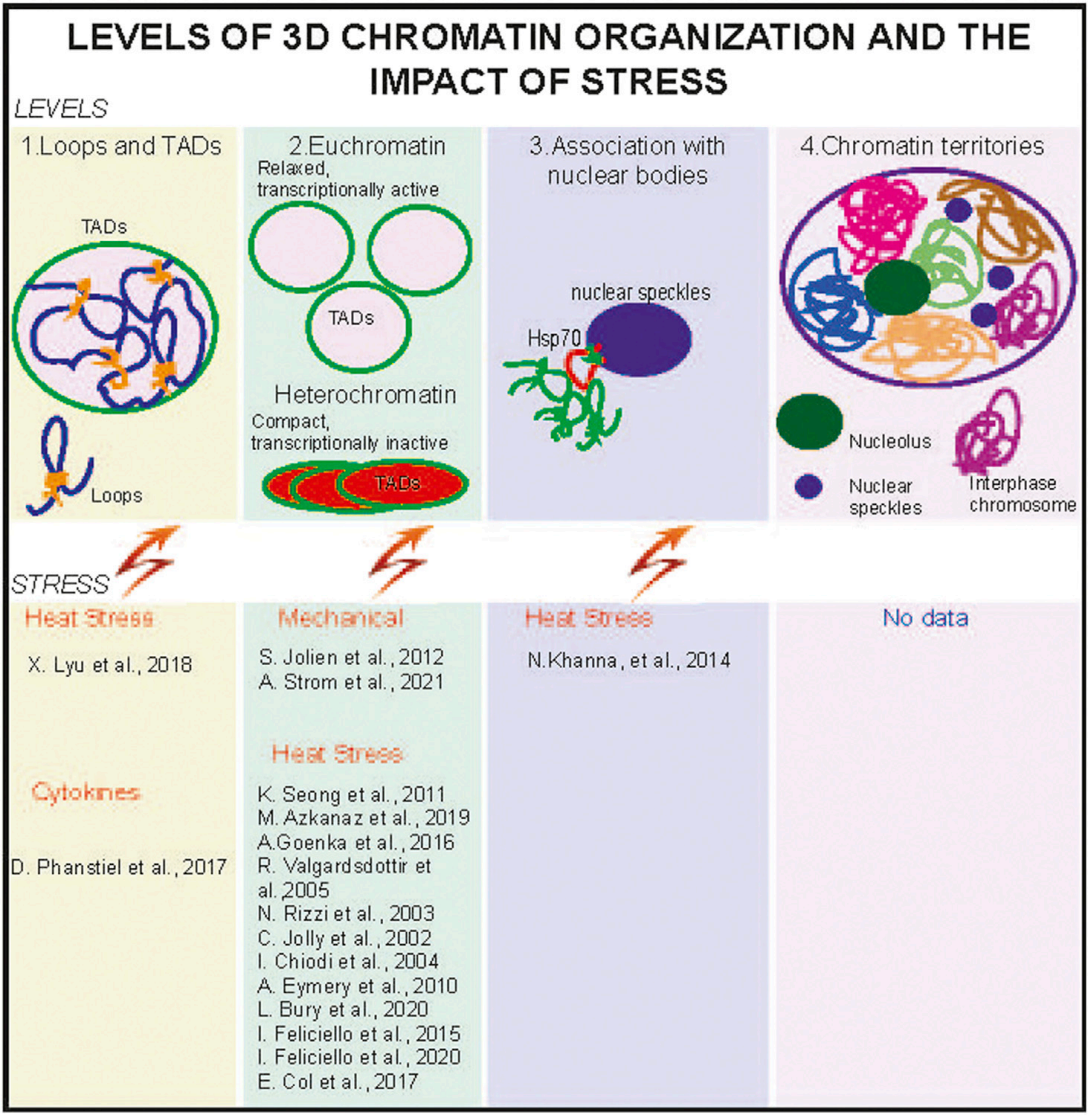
Stress alters 3D chromatin organization at different levels. 3D chromatin is organized at the levels of chromatin loops and topologically associated domains. TADs (1), relaxed and transcriptionally active euchromatin (compartment A), and compact and transcriptionally silenced heterochromatin (compartment B) (2). Euchromatin regions are often found in the nuclear interior and proximal to nuclear speckles (3). Heterochromatin preferentially localizes at the nuclear and nucleolus periphery. Individual chromosomes form chromosome territories. CT, in interphase nuclei (4), and no data about the stress effects on CTs are available. TADs, heterochromatin, and euchromatin were reported to be affected by several stresses (lower panels).
